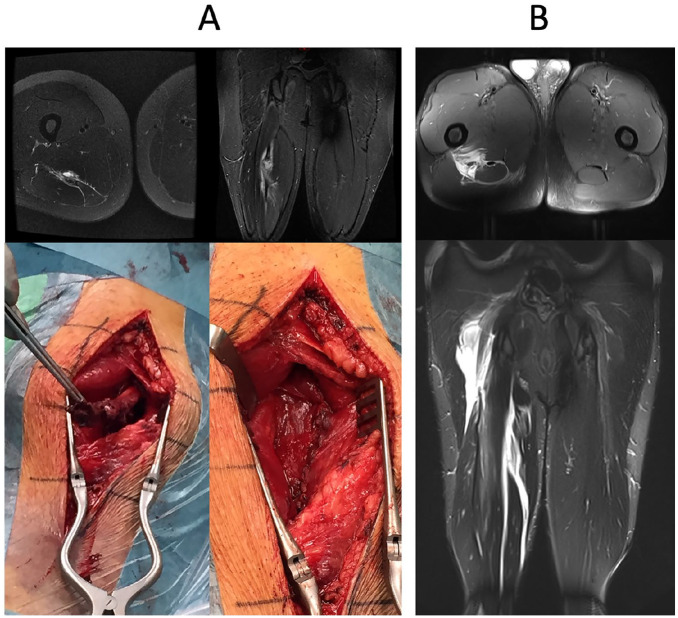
A) Central tendon rupture of the SM (recurrent injury, MRI and perioperative images before and after repair) and B) BF (acute injury, MRI).
